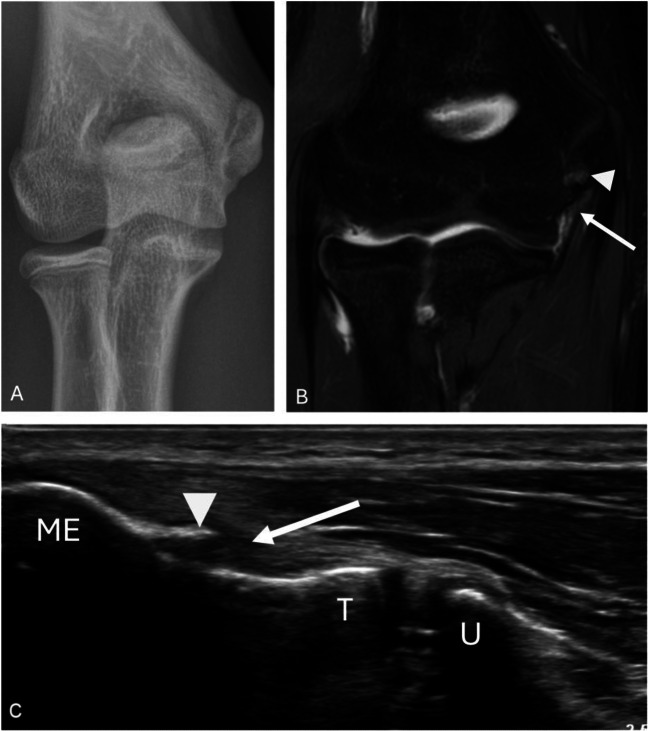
A 13-year-old male baseball pitcher presents with right elbow pain. A Coronal radiograph of the elbow demonstrates no acute osseous abnormality. B Coronal short tau inversion recovery MRI sequence shows a nondisplaced avulsion fracture of the medial epicondyle (*arrowhead*) and a heterogeneous, thickened proximal anterior band of the ulnar collateral ligament with increased intrinsic signal (*arrow*). C Follow-up elbow ultrasound obtained 2 months later demonstrates healing and coalescence of the nondisplaced avulsion fracture (*arrowhead*) with persistent heterogeneity of the proximal ulnar collateral ligament (*arrow*)
